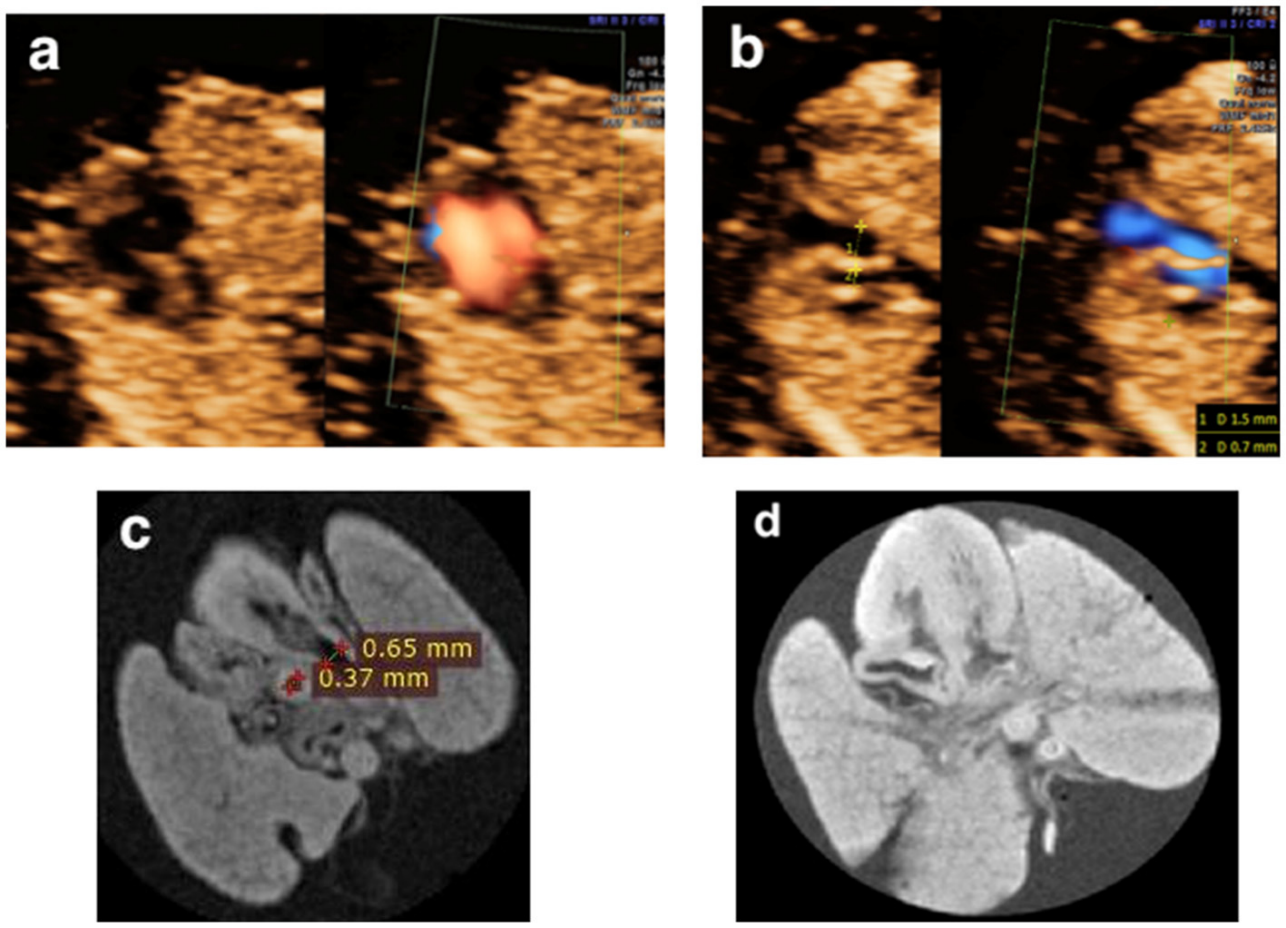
(a,b) A fetus presented with a smaller left ventricle on the four-chamber view and a larger pulmonary artery diameter than that of the aorta (1.5 vs. 0.7 mm) at the three-vessel view in the first trimester and was terminated at 12+6 weeks of gestation for Turner syndrome (Case 12 in Table 2). (c,d) Postmortem MRI showed a normally connected heart with the crossing of the great vessels and a larger pulmonary artery diameter than that of the aorta (0.65 vs. 0.37 mm).
